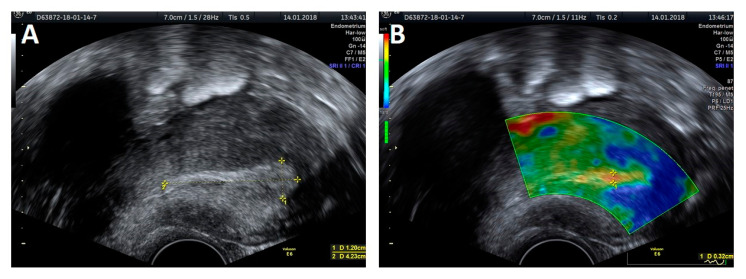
A 2D ultrasound presentation: endometrial thickness (A) and elastography: thickness measurement of the softest layer of the endometrium (B).
